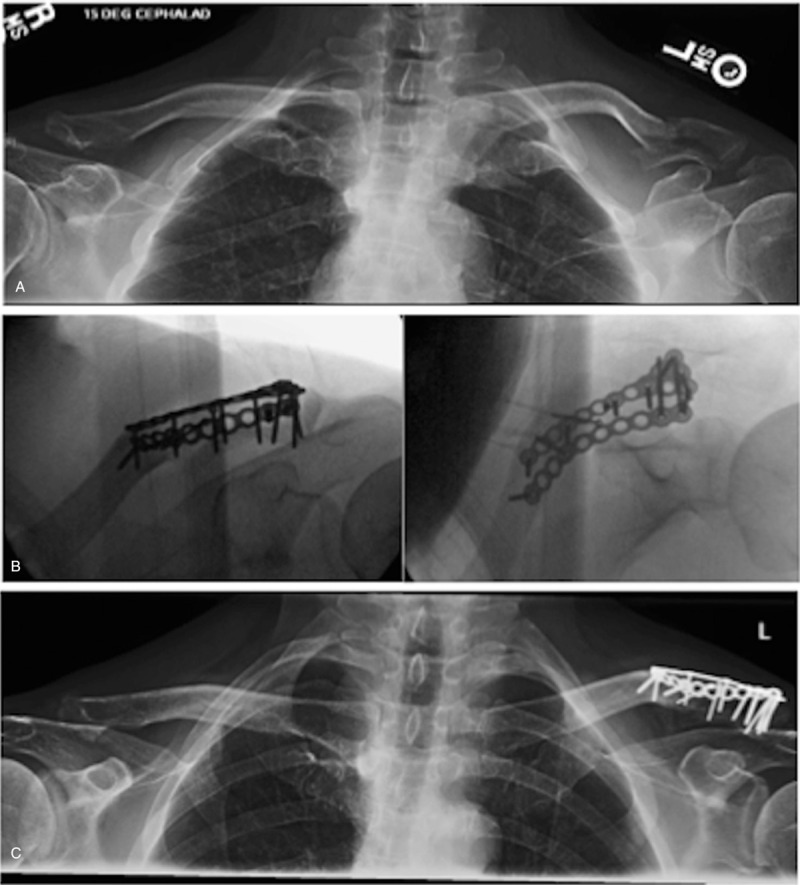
(A) Injury radiographs, (B) intraoperative fluoroscopy, and (C) final postoperative radiographs demonstrating healing of a Neer type-V distal clavicle fracture fixed with dual mini-fragment plates. Multiple lag screws were placed into the inferior fragment attached to the coracoclavicular ligaments. Note the interdigitating screws placed through orthogonal plates to enhance fixation in the distal segment.
